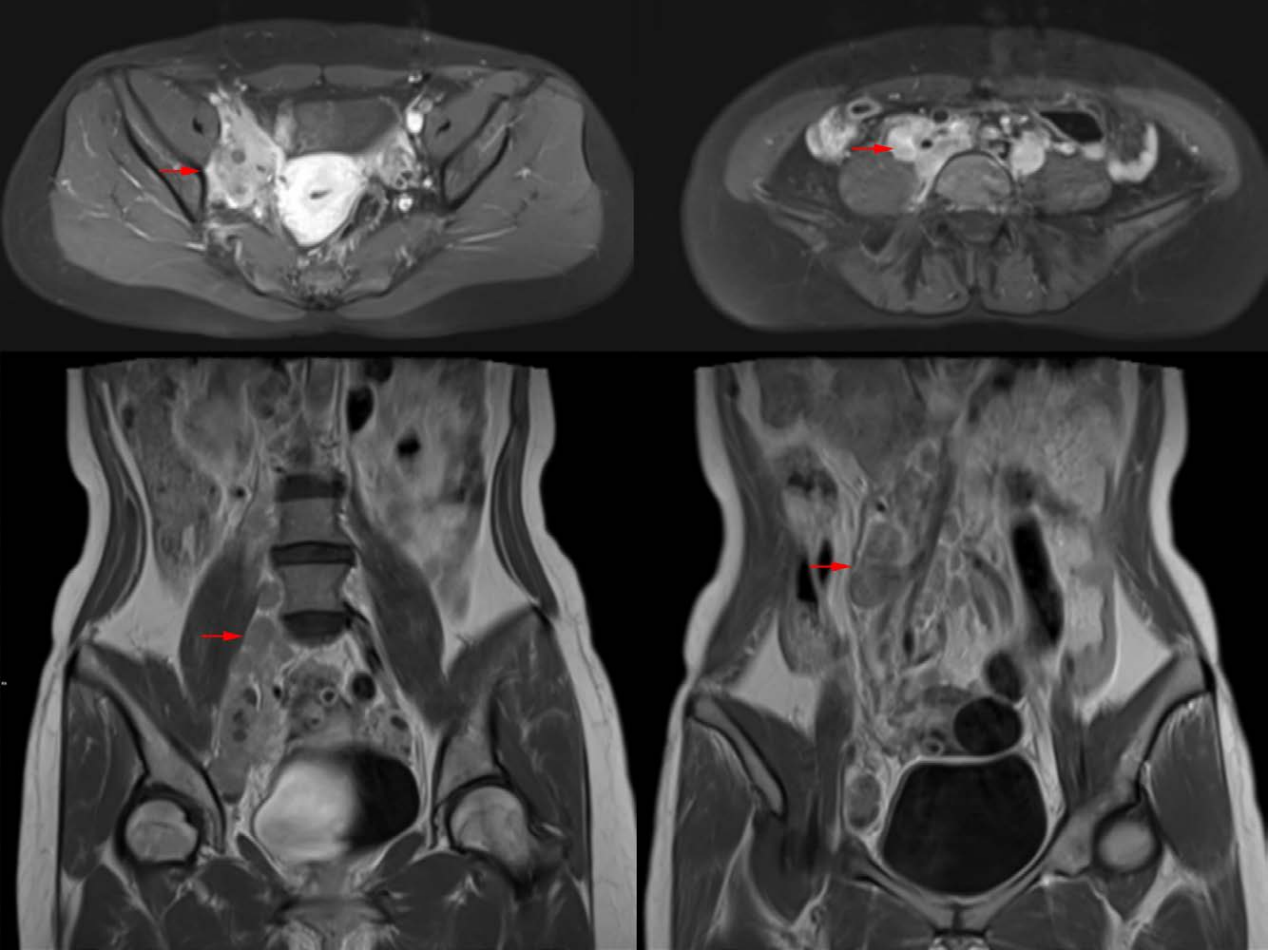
Magnetic resonance imaging (MRI) with contrast findings.
